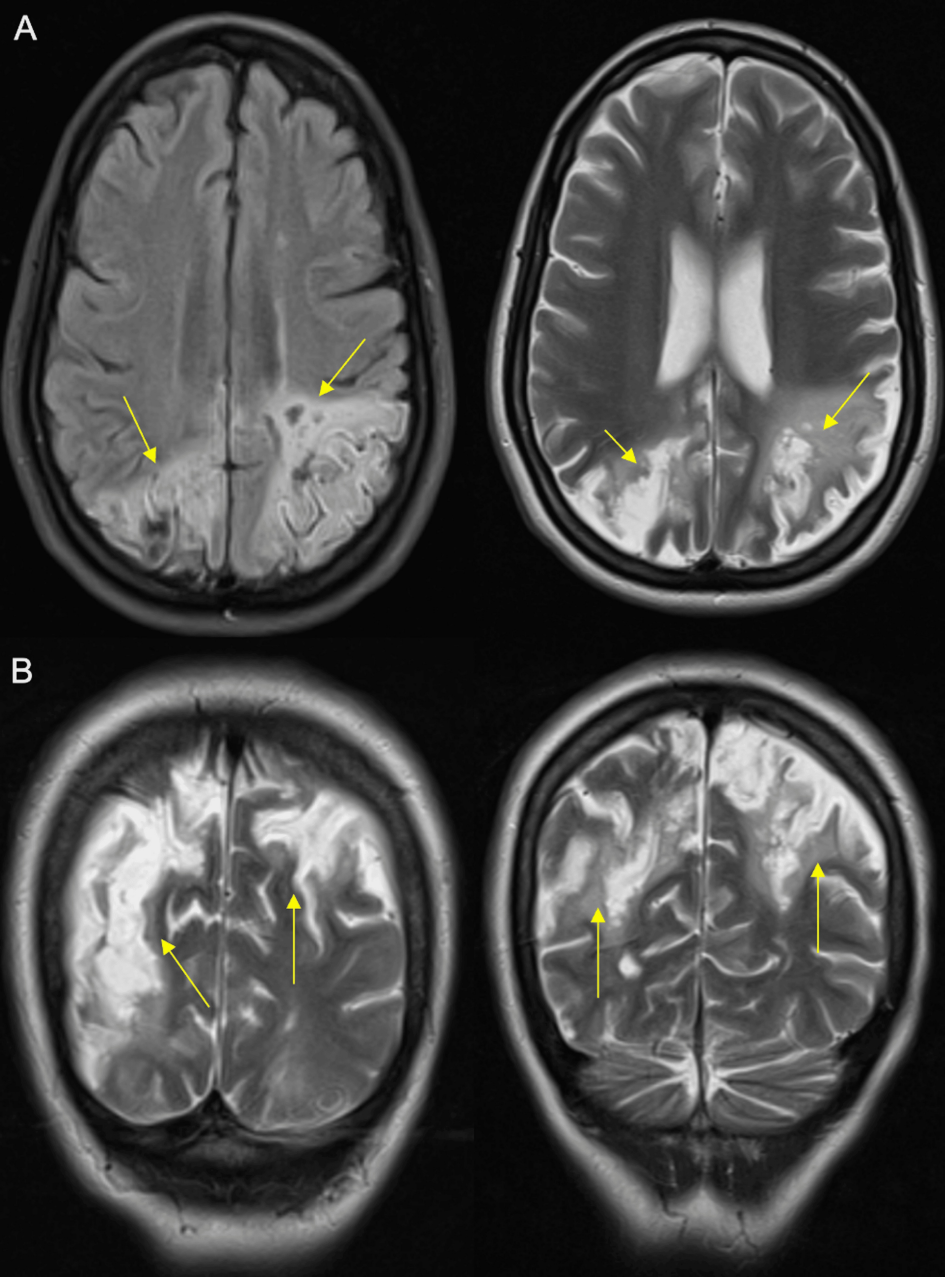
Brain MRI - revaluation T2-weighted images. Panel A: axial plane. Panel B: coronal plane. Yellow arrows pointing to the lesion areas MRI: magnetic resonance imaging
